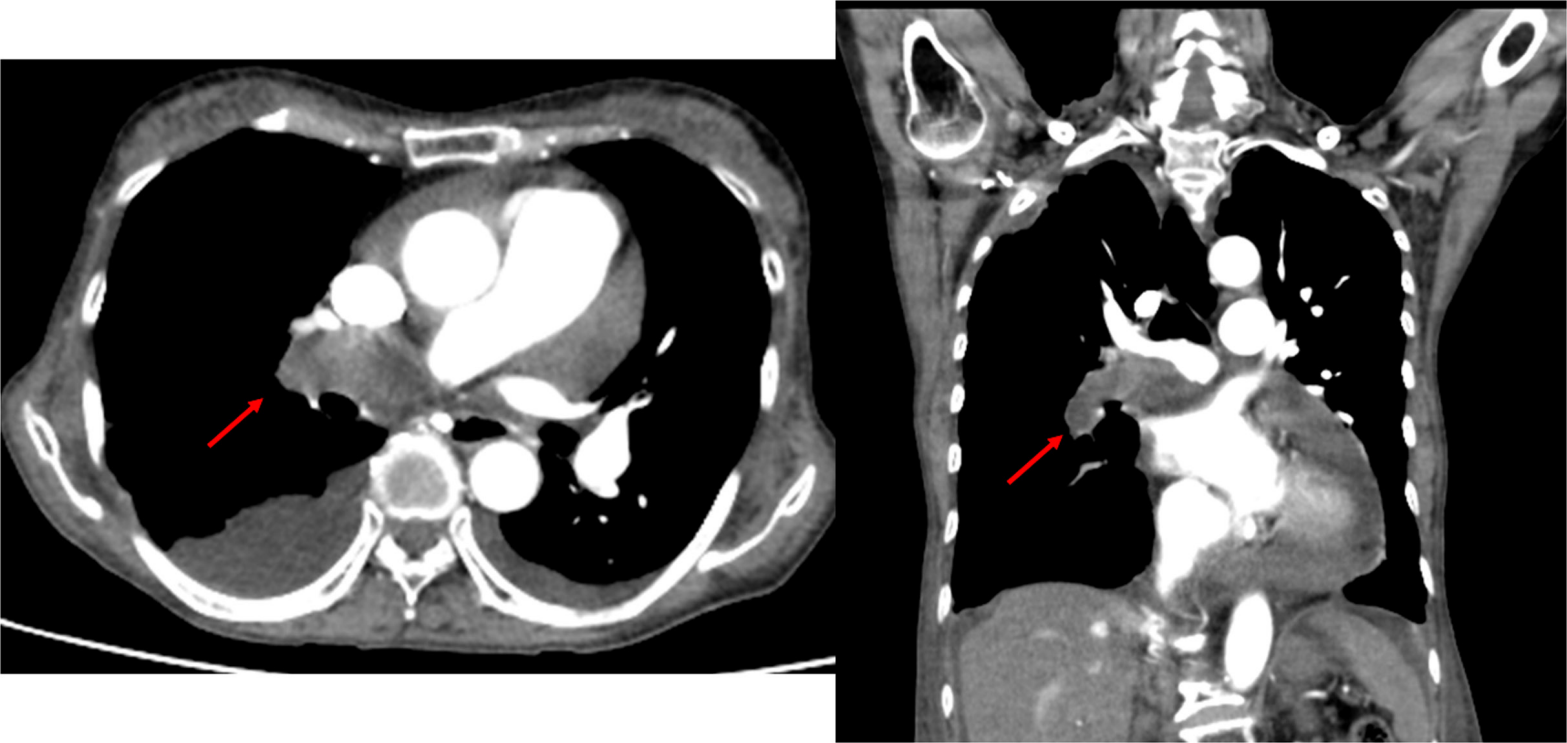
Thoracic contrast‐enhanced computed tomography showing a space‐occupying lesion, considered to be a pulmonary thrombus (red allow).
